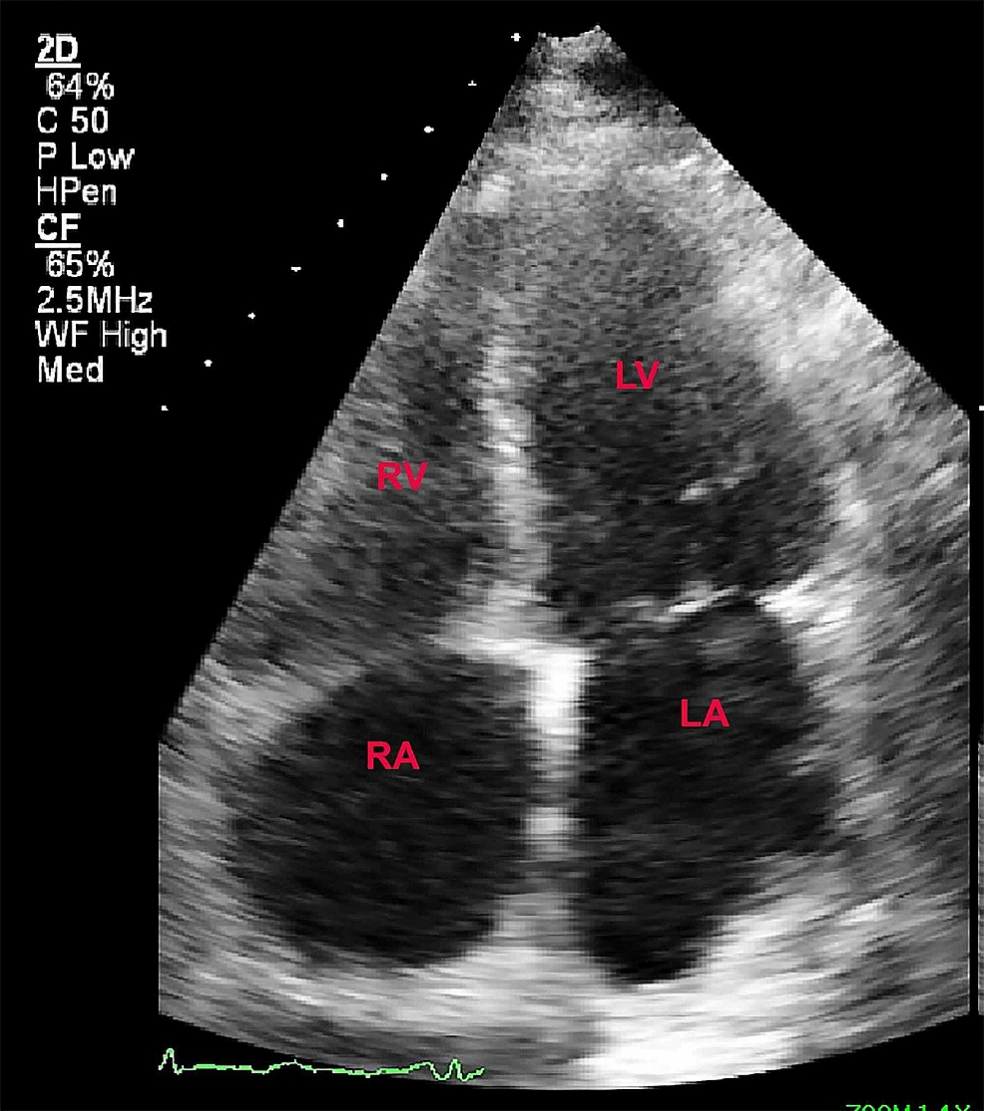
Transthoracic echocardiogram revealed biatrial enlargement.
